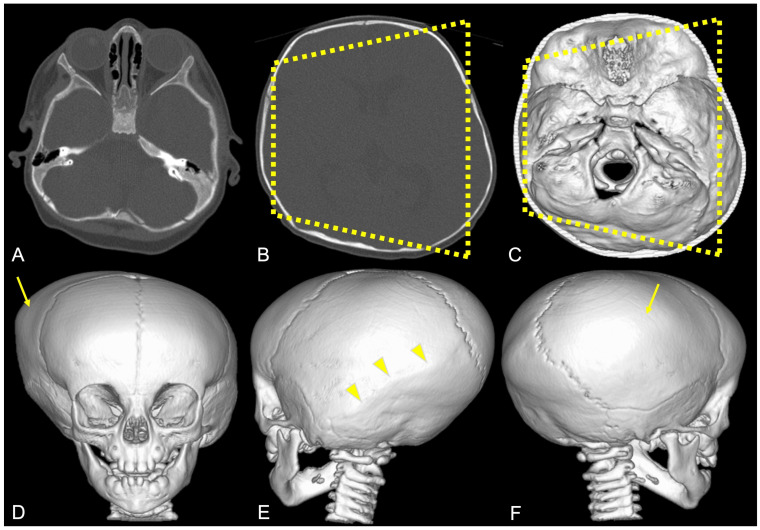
Example of high-resolution three-dimensional CT images (A,B) of the skull with volumetric image reconstruction (C–F) in a patient diagnosed with posterior plagiocephaly due to right-sided lambdoid synostosis (yellow arrowheads), with a trapezoidal shape of the head (dotted yellow line) and compensatory left-sided frontal–parietal bossing (yellow arrow).
